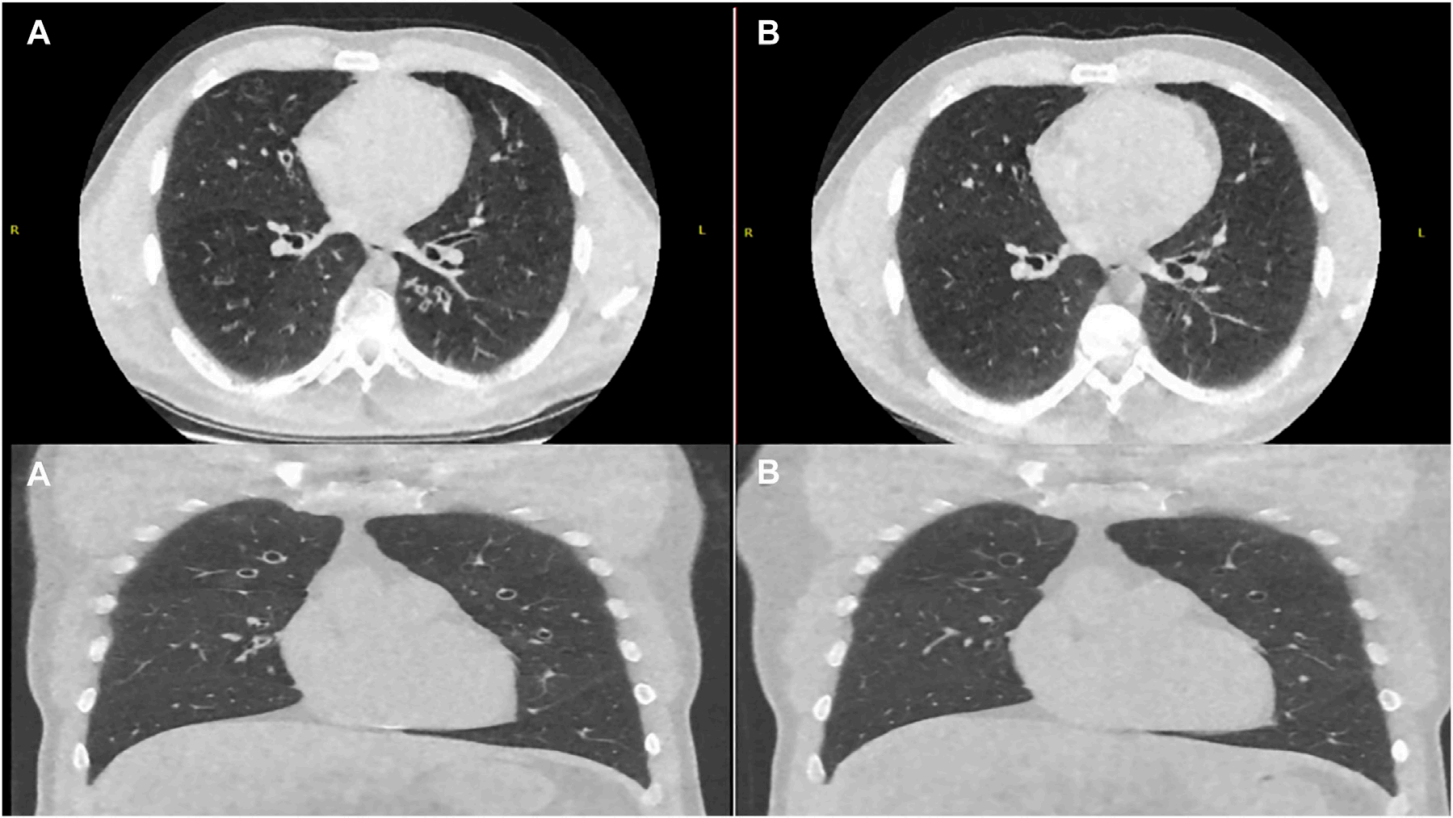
Axial and Coronal Ultra-Low Dose CT Thorax pretreatment (A) and post modified dose regimen (B) showing reduction in the degree of bronchial wall thickening and mucoid impaction.
